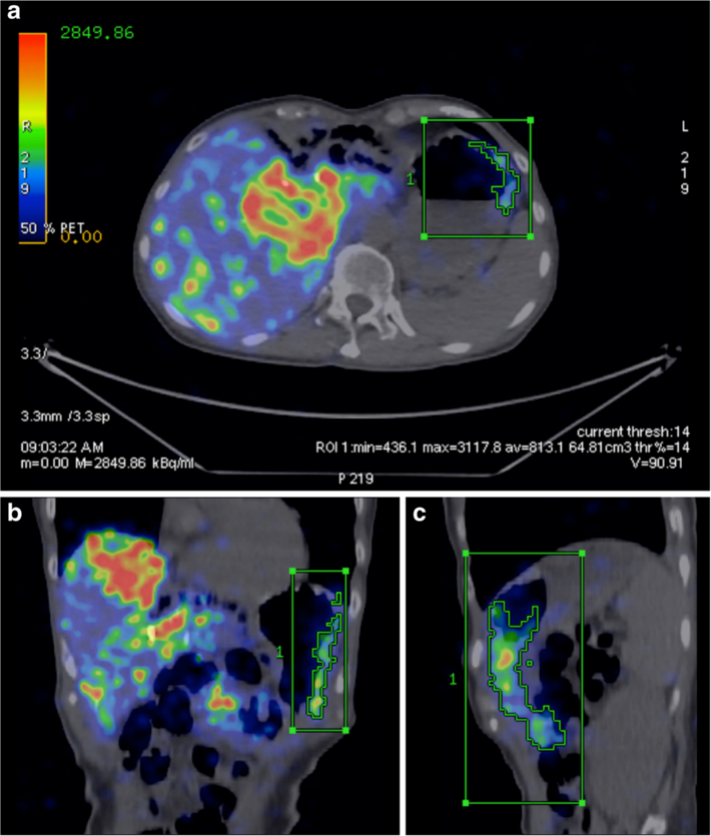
Patient 17. Non-target ^90^Y activity along the gastric greater curve causing CTCAE grade 3 toxicity [1]. A ^90^Y PET VOI of the activity along the gastric greater curve was defined by volumetric isocontour thresholding, visually constrained to its CT anatomical margins, shown here in (a) trans-axial, (b) coronal, and (c) sagittal planes. Within this VOI, the mean ^90^Y radioconcentration at the time of scan (813.1k Bq/ml) was decay-corrected back to the time of ^90^Y radioembolization (1,021.5 kBq/ml). By ^90^Y MIRD macrodosimetry, the non-target mean absorbed dose to the gastric greater curve was approximately 49 Gy within the VOI. Gastroscopy and biopsy findings were reported in part 1 [1].
